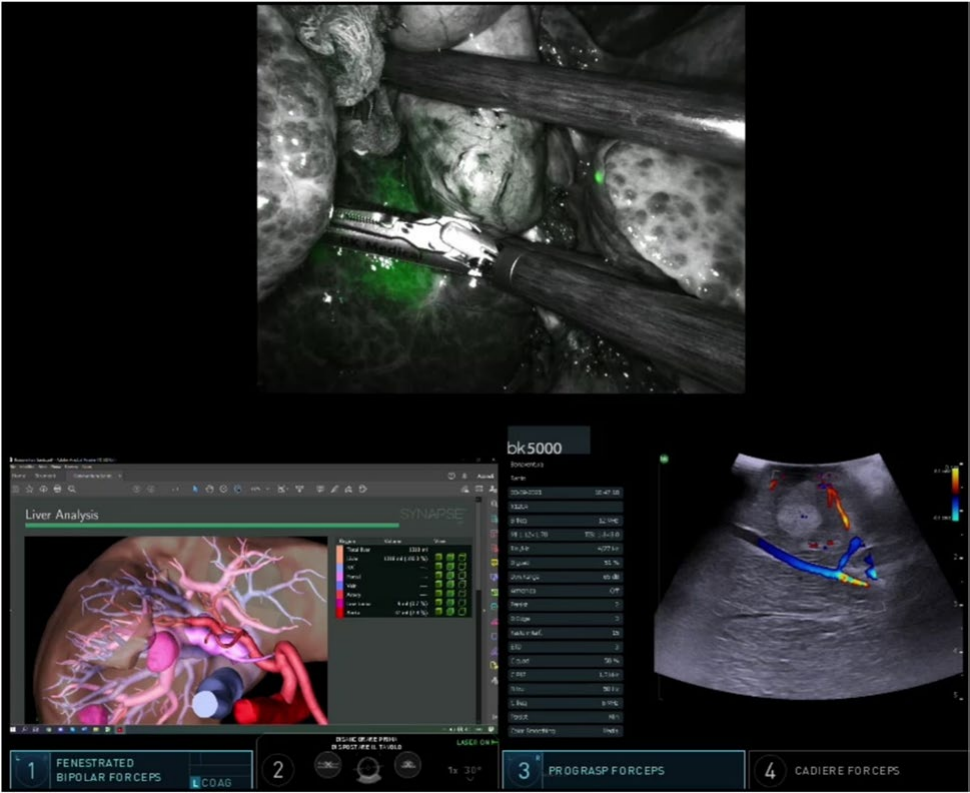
The integrated robotic platform permits the simultaneous visualization of several systems together with the operating field, such as radiologic images, 3D reconstructions, and intraoperative ultrasounds. This function can reduce workflow disruption and provide instant comparison between multiple modalities
